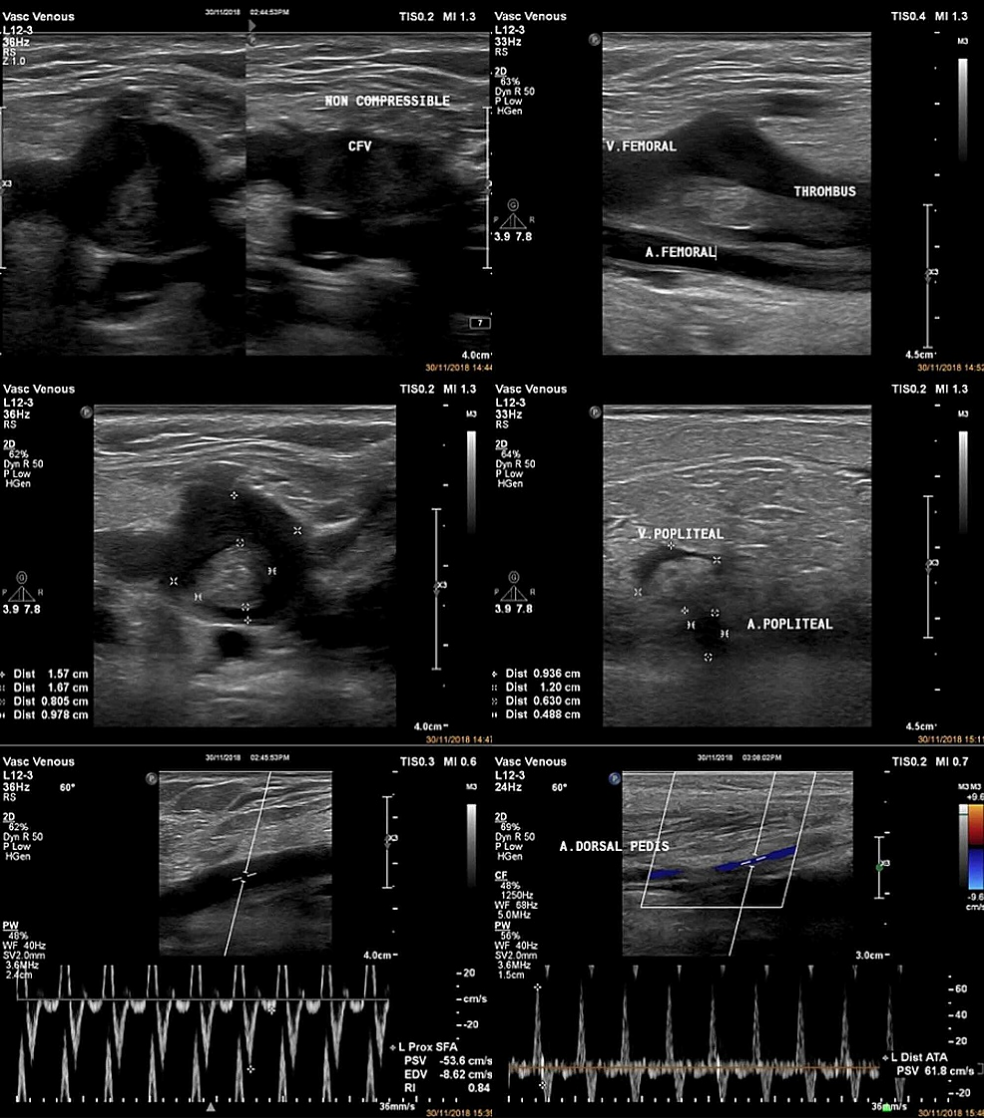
Duplex ultrasound of left lower extremity showed large thrombus located from proximal part of common femoral vein to popliteal vein, along with no flow and non-compressible vein. These findings were consistent to DVT of the leg. Normal flow was noticed throughout arterial systems. DVT: Deep vein thrombosis
